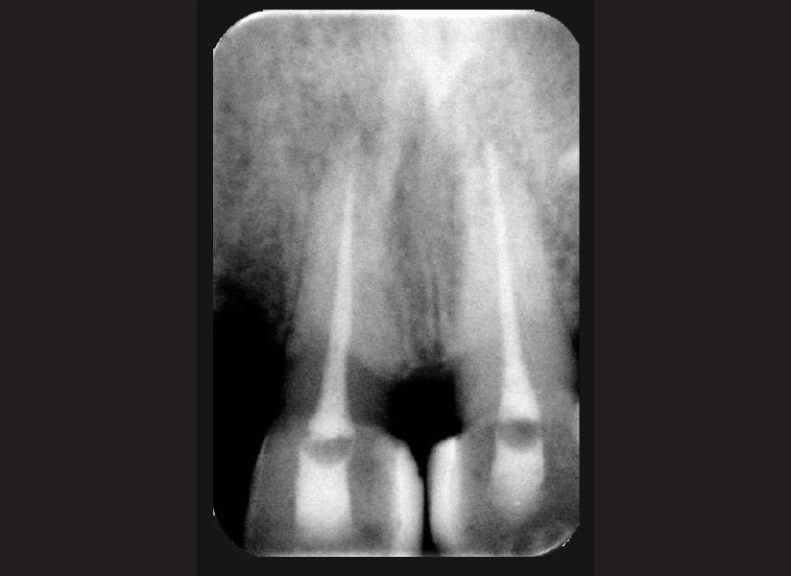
IOPA radiograph at 1-year follow up
